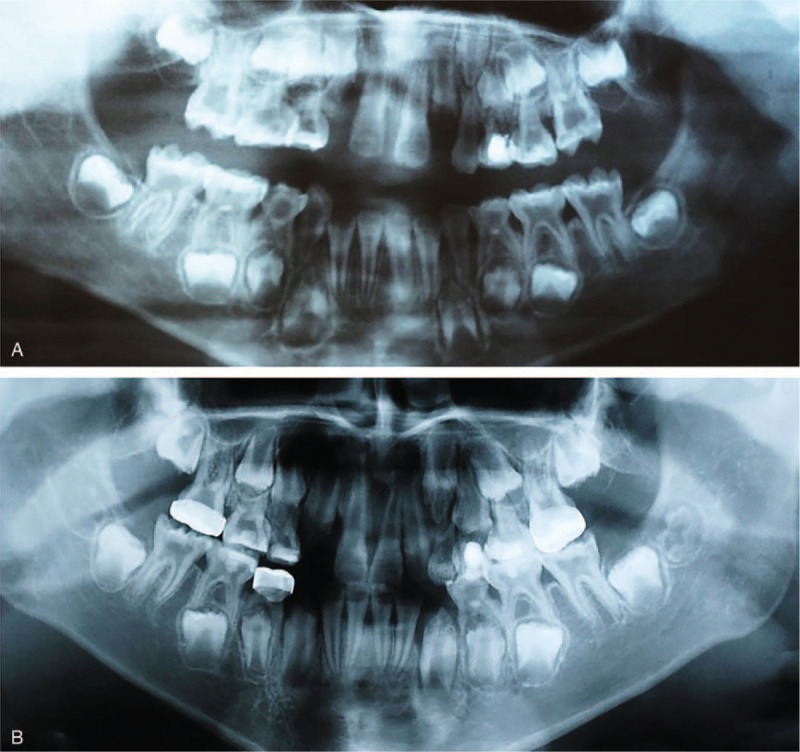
Panoramic radiograph. (A) The panoramic radiograph image of the initial clinical examination. (B) The panoramic radiograph image after 3 years of dental intervention shows the growth of tooth germs.
